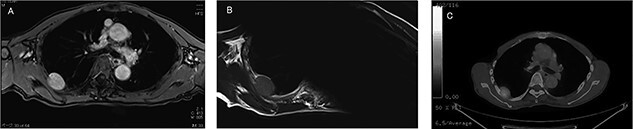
Preoperative MRI and PET-CT: (A) contrast-enhanced T1WI: hyper-intense; (B) T2WI: iso-intense; (C) PET-CT: SUVmax 3.4–4.1.
